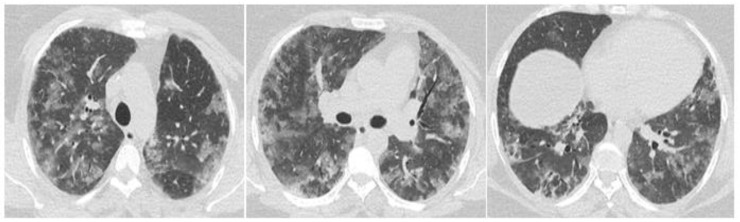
The chest CT scan performed at the time of sudden respiratory degradation, shows a rapidly progressive interstitial lung disease with the appearance of diffuse and extensive ground glass opacities.
